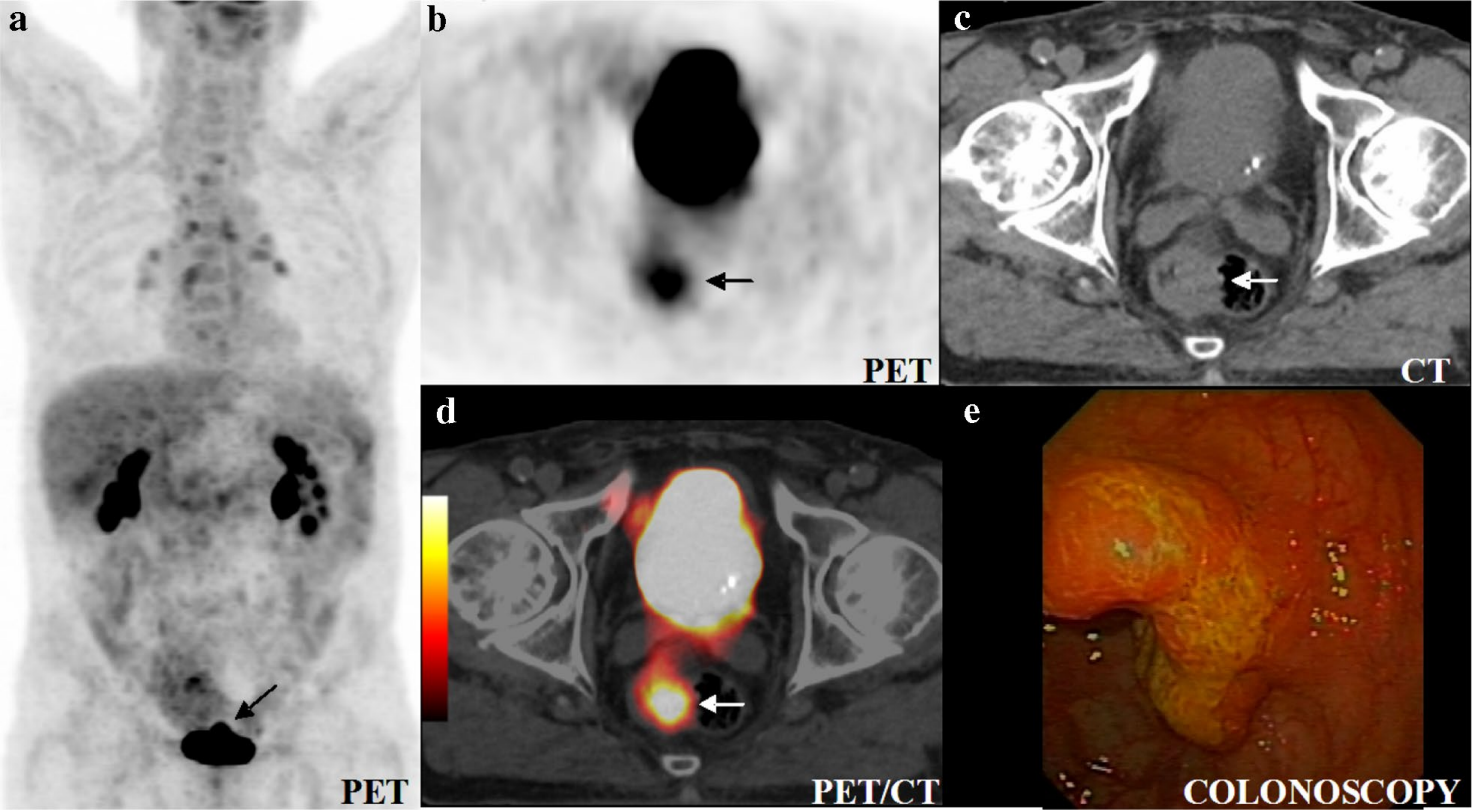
Colorectal cancer (*arrow*) in the initial staging. Maximum intensity projection (MIP) of PET captured in the coronal projection of the 360°-rotation (a), axial slices of PET (b), CT (c) and PET/CT (d) and colonoscopy (e). As in Fig. 2, rotating the maximum intensity projection (MIP) or scrolling through axial PET slices (b) is mandatory to detect carcinomas behind the urinary bladder. PET clearly depicts the colorectal cancer, here with an SUV_max_ of 6.1
